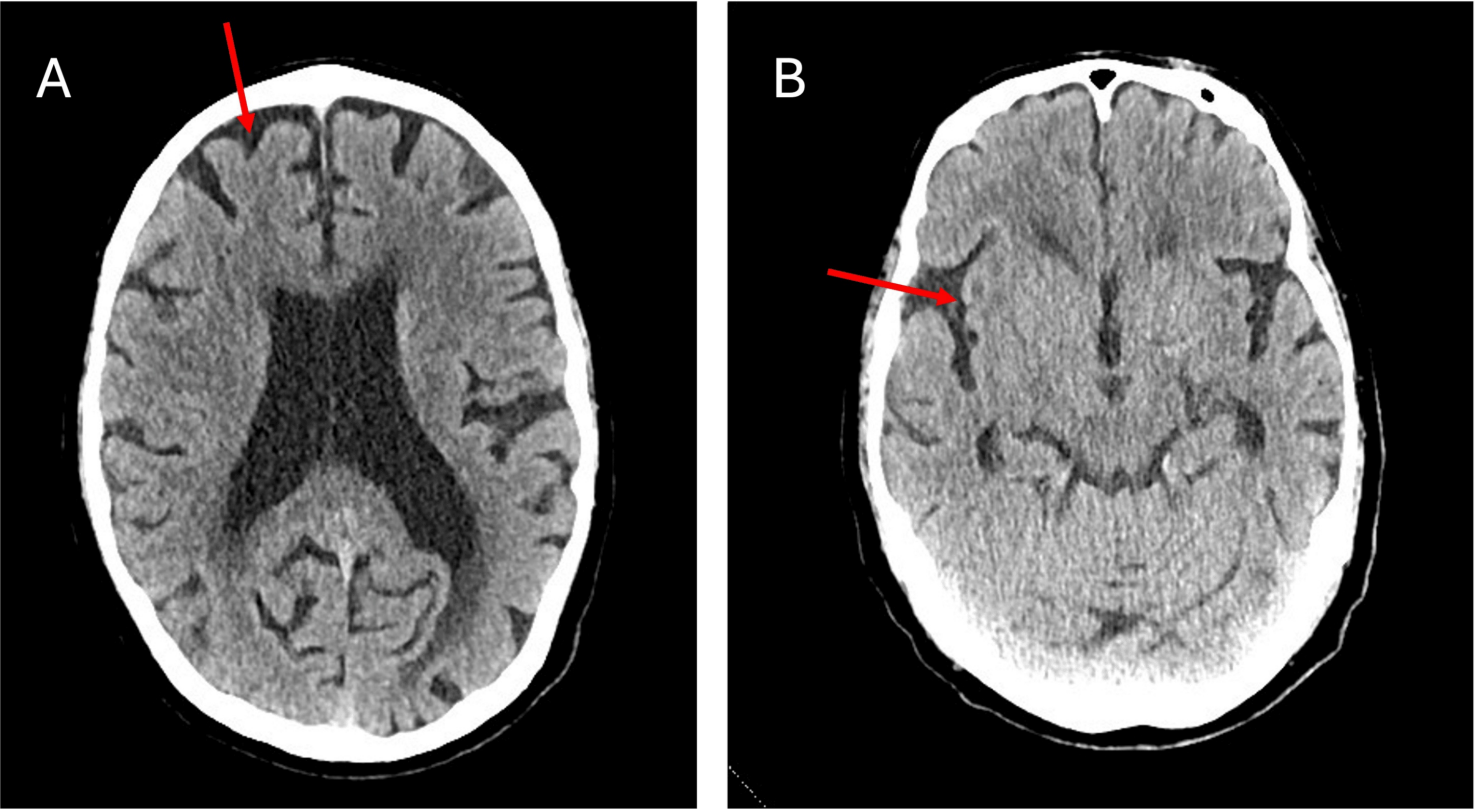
Computed tomography of the head without contrast on admission. A) The arrow indicates a deep sulcus. B) The arrow indicates an area of hyperintensity.
